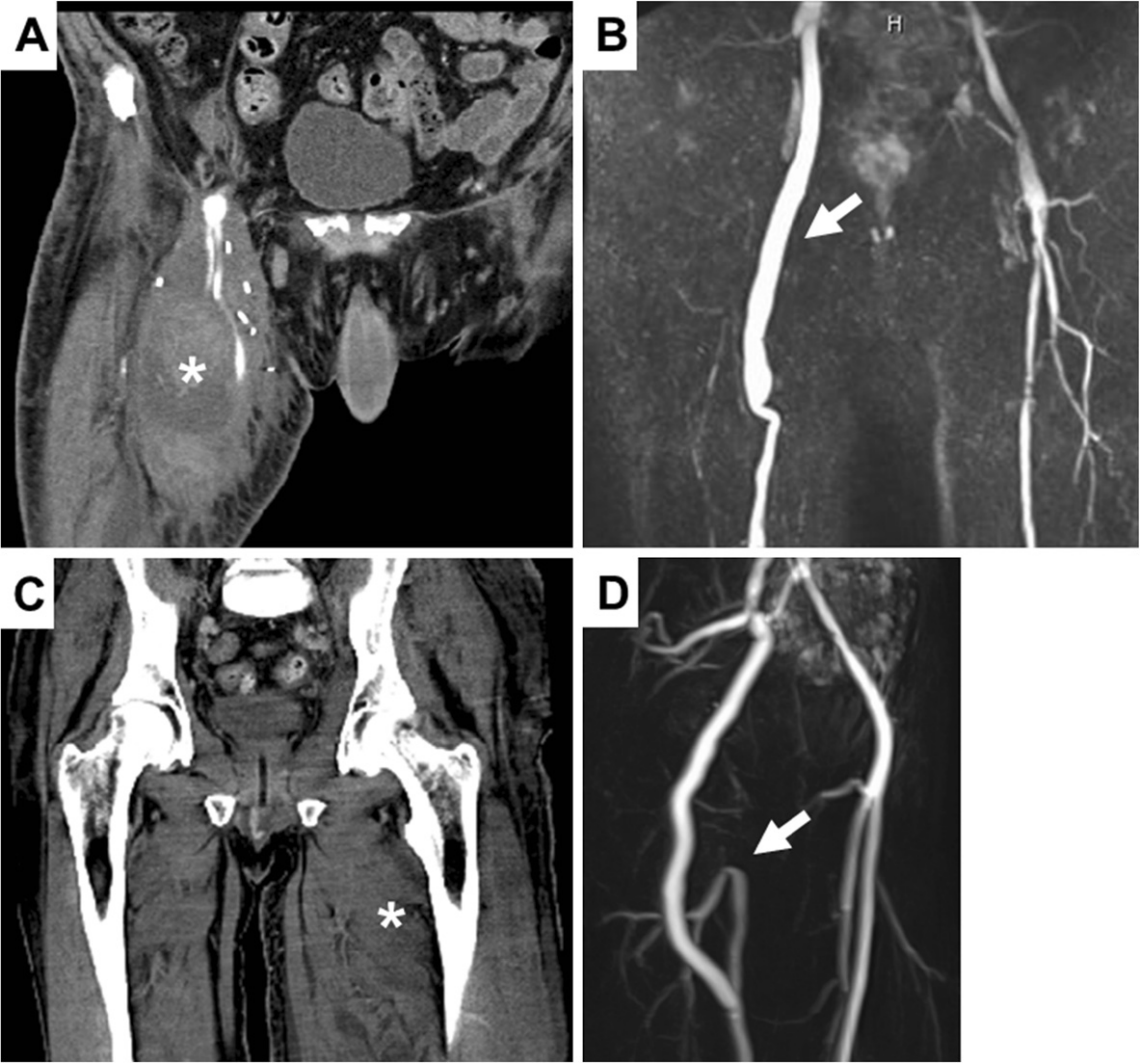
(A) Computed tomography scan of case 1 obtained after rebleeding due to septic pseudoaneurysm showing liquid, putrid formation (asterisk) with arterial extravasation caused by erosion of the distal anastomosis of the greater saphenous vein interposition and extensive perifocal soft-tissue edema in the right upper thigh. (B) Magnetic resonance angiogram of the obturator bypass in case 1 nearly 3 years after bypass surgery showing bypass perfusion free of stenosis (arrow). Case 2 (C) Computed tomography scan of case 2 at time of admission showing swelling, subcutaneous purulent formation and perifocal edema (asterisk). (D) Magnetic resonance angiogram obtained 3 months after surgery in case 2 showing the obturator bypass with retrograde perfusion of the profundal femoral artery by additional end-to-end anastomosis with proximal superficial femoral artery (arrow).
